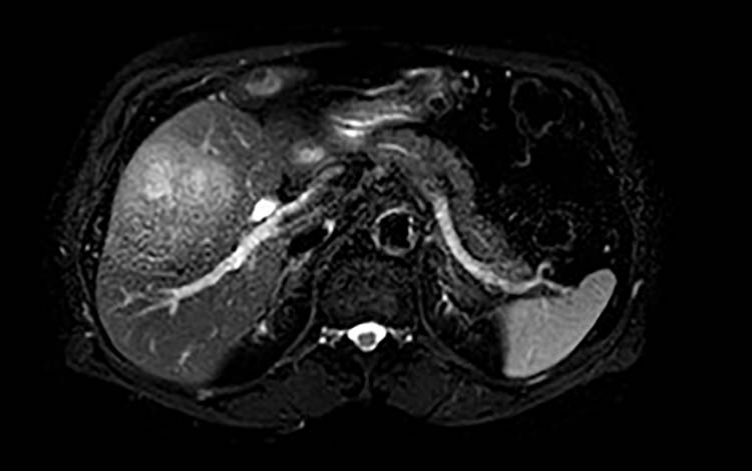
MRI (axial view): 7.1 × 8.1 cm mass originating from the gallbladder involving segments IVb, V, VI with areas of peripheral enhancement and central necrosis.
